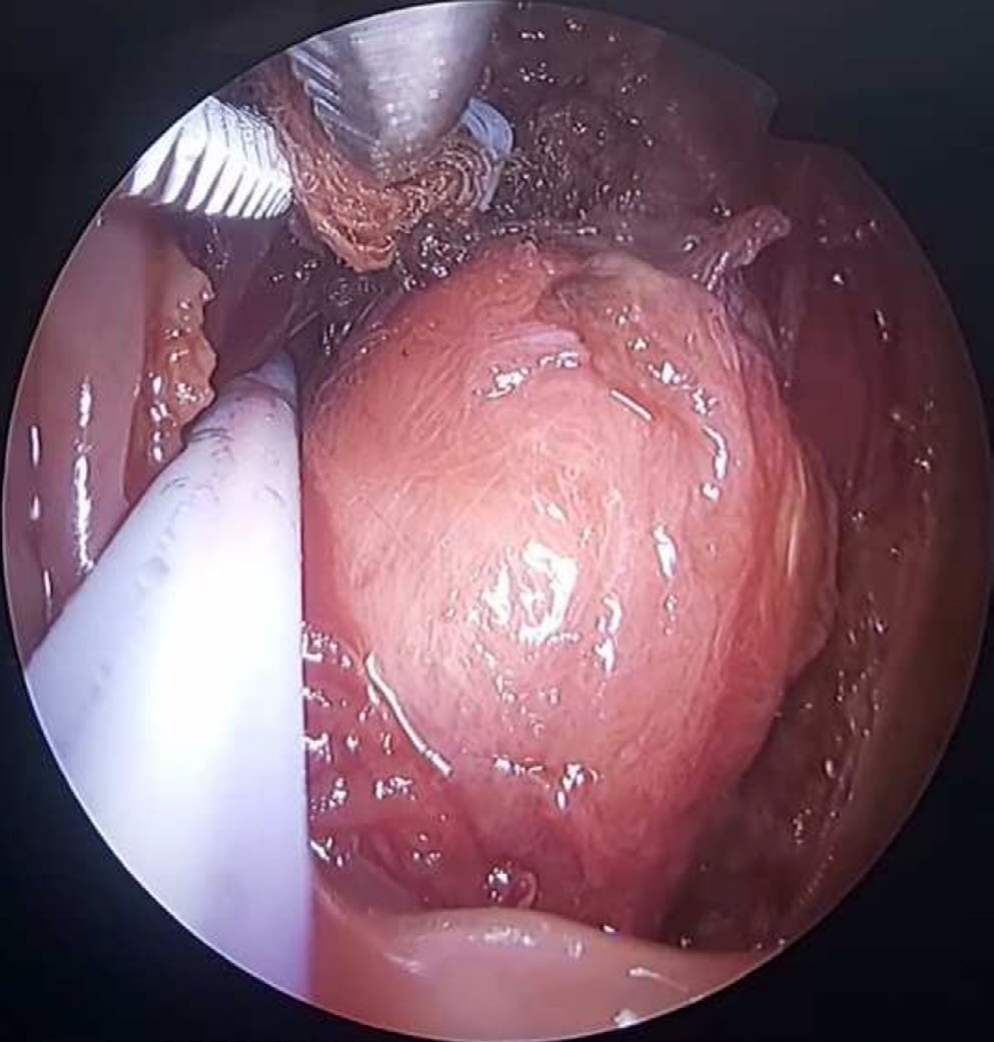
Intraoperative view of the mass and use of Doppler to identify internal carotid artery
